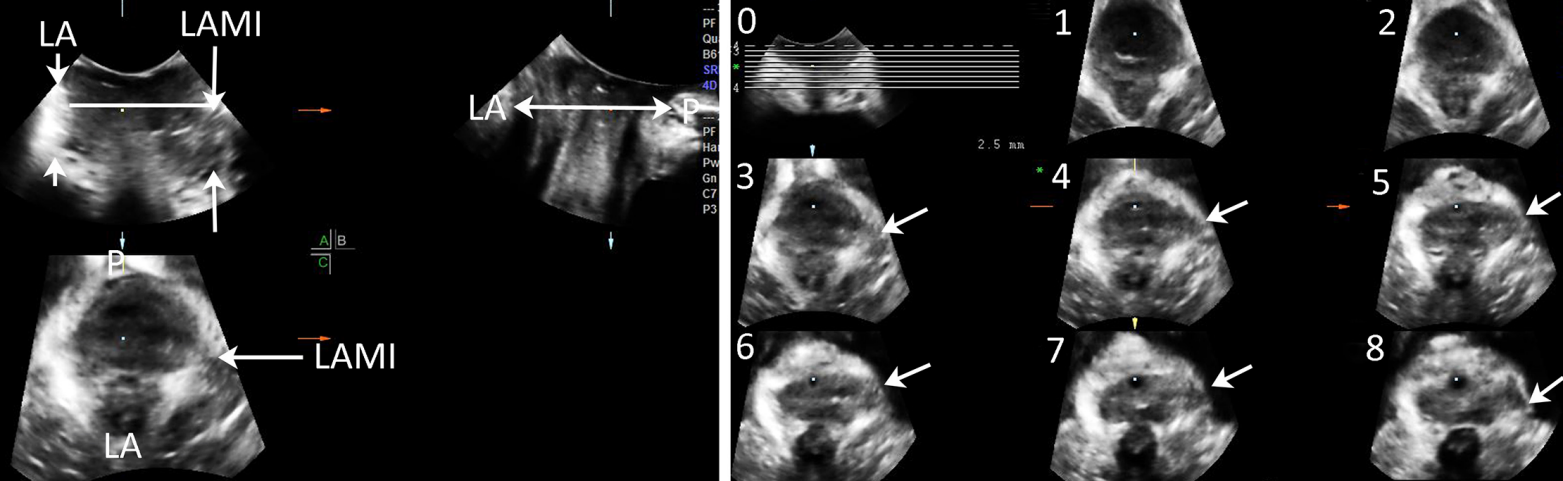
Left image depicts an intact levator ani (LA) muscle on right side (short white arrows) and a left-sided avulsion in coronal plane (long white arrows), and the measurement of the levator ani muscle injury (LAMI) depth (long white arrows) above the minimal axial plane in the coronal plane (reference line is the white horizontal line). Right image depicts levator avulsion on the left side in slices 3–8 on tomographic ultrasound imaging (TUI). TUI score of the right side is 0 and 6 on the left side. P: symphysis pubis.
